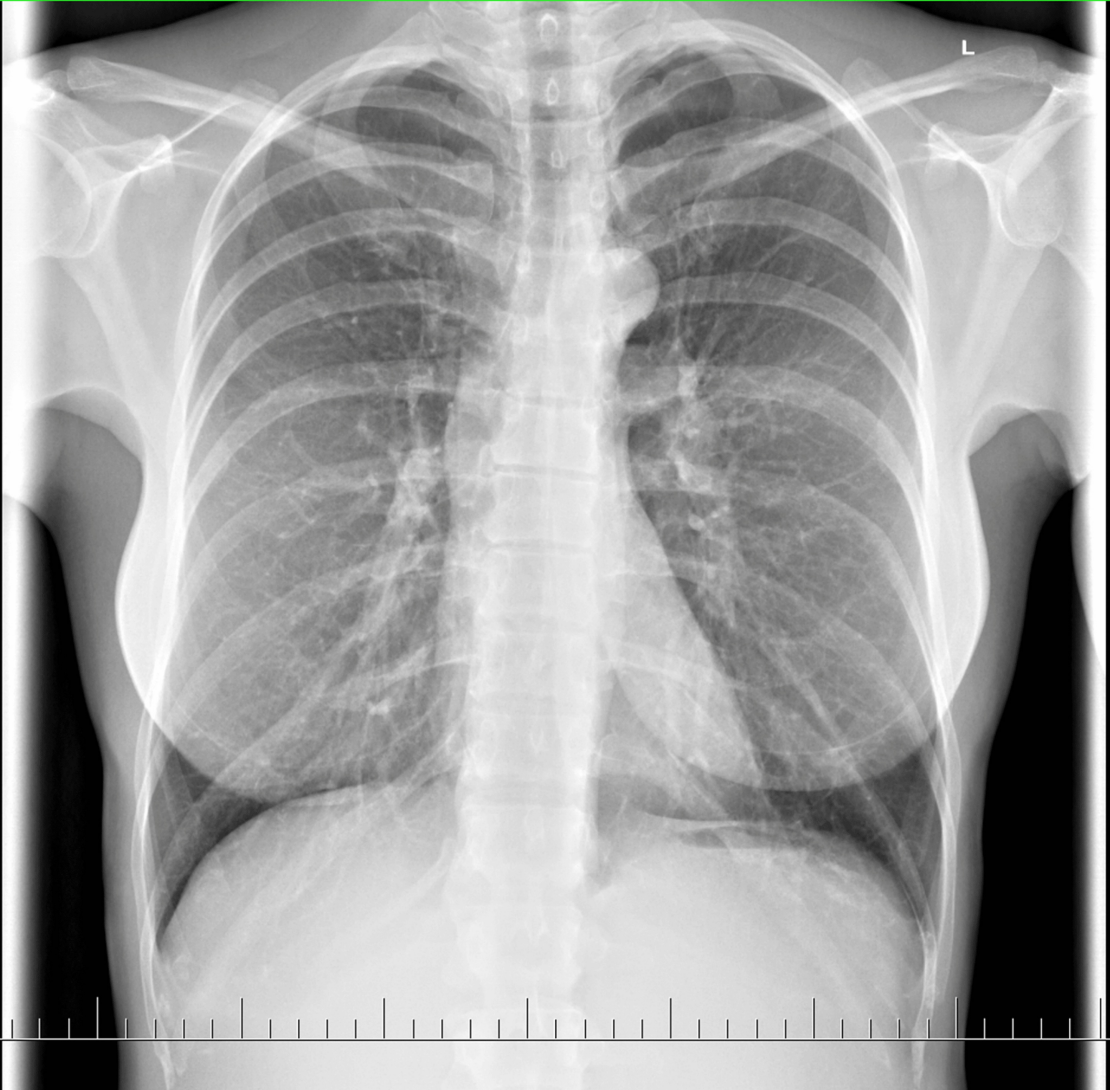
Chest X-ray after six months Chest X-ray showing no significant findings.
